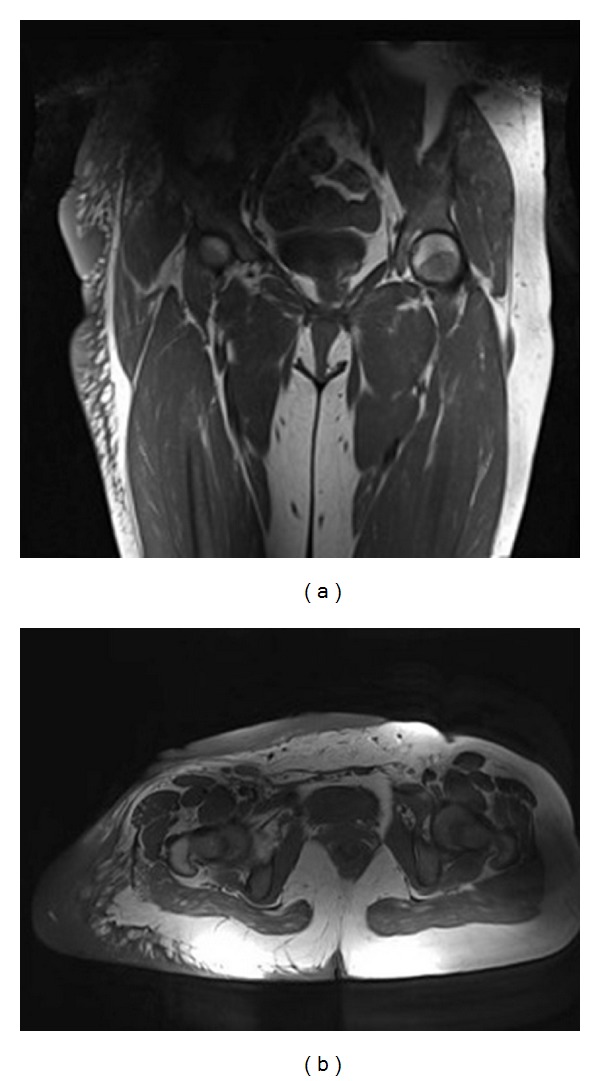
Postoperative MRI images (2-year followup). Not recurrence or tumor progression.
